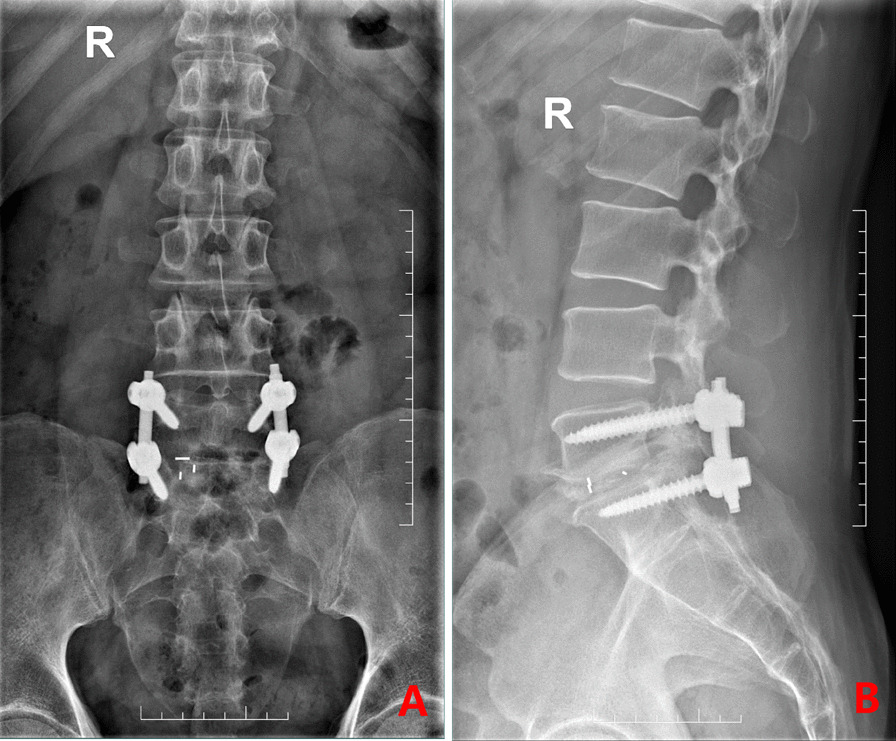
Postoperative follow-up radiography. A Postoperative follow-up anteroposterior X-ray image. B Postoperative lateral x-ray image taken on follow-up at the 3rd postoperative month. The satisfactory position of the internal fixation device between the 5th lumbar and the 1st sacral vertebral bodies can be seen together with the satisfactory fusion of the two vertebrae
